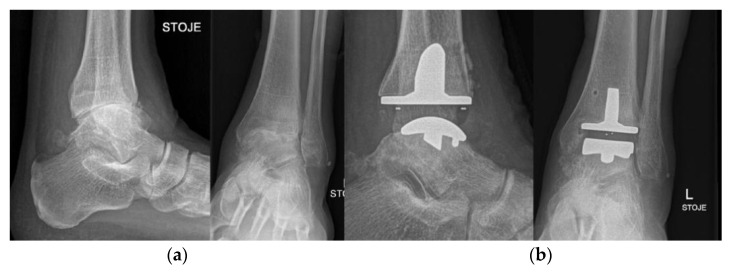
Total ankle arthroplasty (TAA). (a) preoperative X-ray; (b) postoperative X-ray after total ankle arthroplasty.
